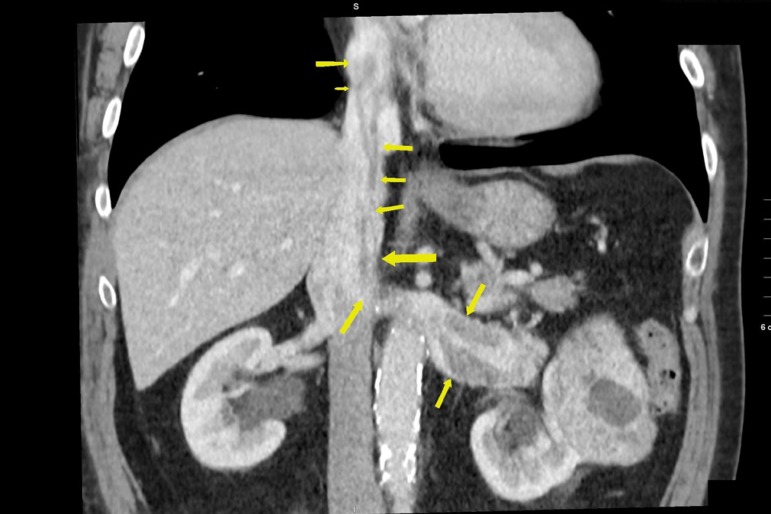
CT scan of male patient with left kidney tumor and thrombus propagation
